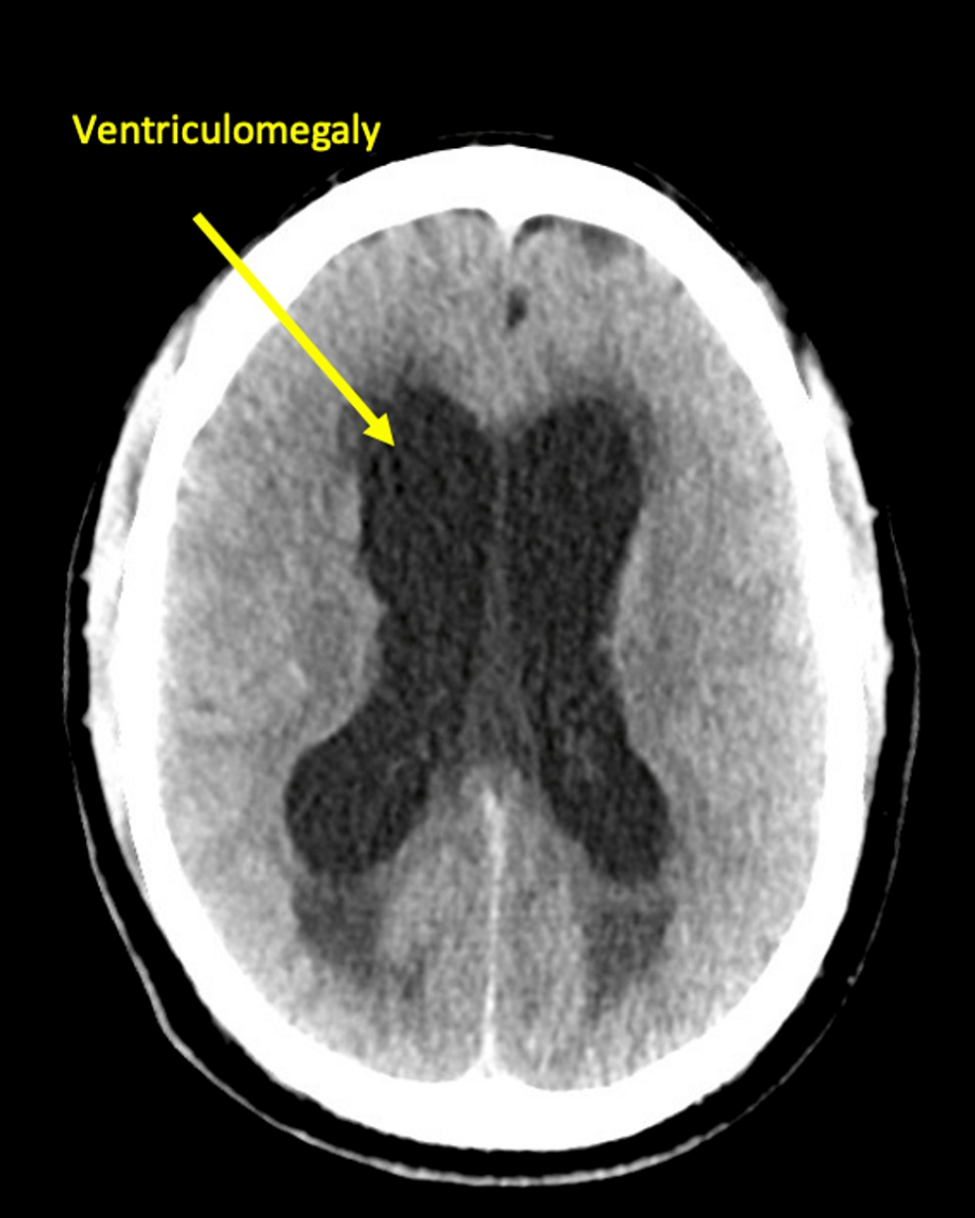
CT head without contrast on day 10 of admission revealed worsening ventriculomegaly with some transtentorial mass effect and signs of suspected cerebral edema.
